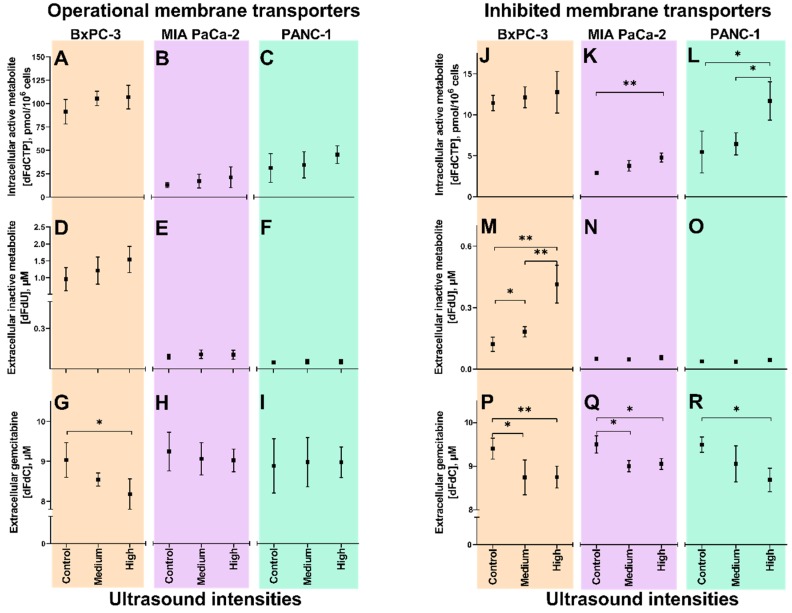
Gemcitabine uptake and metabolism following sonoporation of pancreatic ductal adenocarcinoma (PDAC) cell lines with operational and inhibited membrane transporters. Extracellular concentrations of gemcitabine (dFdC, panels G, H, I and P, Q, R), extracellular inactive metabolite (dFdU, panels D, E, F and M, N, O) and intracellular active metabolite (dFdCTP, panels A, B, C and J, K, L) in BxPC-3 (orange), MIA PaCa-2 (purple) and PANC-1 (green). Results displayed as mean±SD (*n* = 3–4). * *p* < 0.05,  *p* < 0.01 (Unpaired students *t*-tests). Notice the different scales on the *Y*-axes of extracellular dFdU and intracellular dFdCTP concentrations in experiments with operational vs. inhibited membrane transporters. “Operational membrane transporters” (panels A–I): 60 min incubation with 10 µM gemcitabine, 3.84 × 10^6^ ppmL Sonazoid^®^ microbubbles and 5 min ultrasound (US) at two acoustic intensities (Medium^1^, High^2^) and no US (Control), in Petakas. “Inhibited membrane transporters” (panels J–R): 20 min pre-incubation with 100 µM dilazep followed by 60 min incubation with 10 µM gemcitabine, 3.84 × 10^6^ ppmL Sonazoid^®^ microbubbles and 5 min US at two acoustic intensities (Medium^1^, High^2^) and no US (Control), in Petakas. ^1^ Medium US intensity: 2.0 MHz, MI 0.2, 80 cycles, DC 1.8%, *I*_SPPA_ 3 W/cm^2^ and *I*_SPTA_ 50 mW/cm^2^; ^2^ High US intensity: 2.0 MHz, MI 0.378, 160 cycles, DC 3.6%, *I*_SPPA_ 10 W/cm^2^ and *I*_SPTA_ 358 mW/cm^2^.
